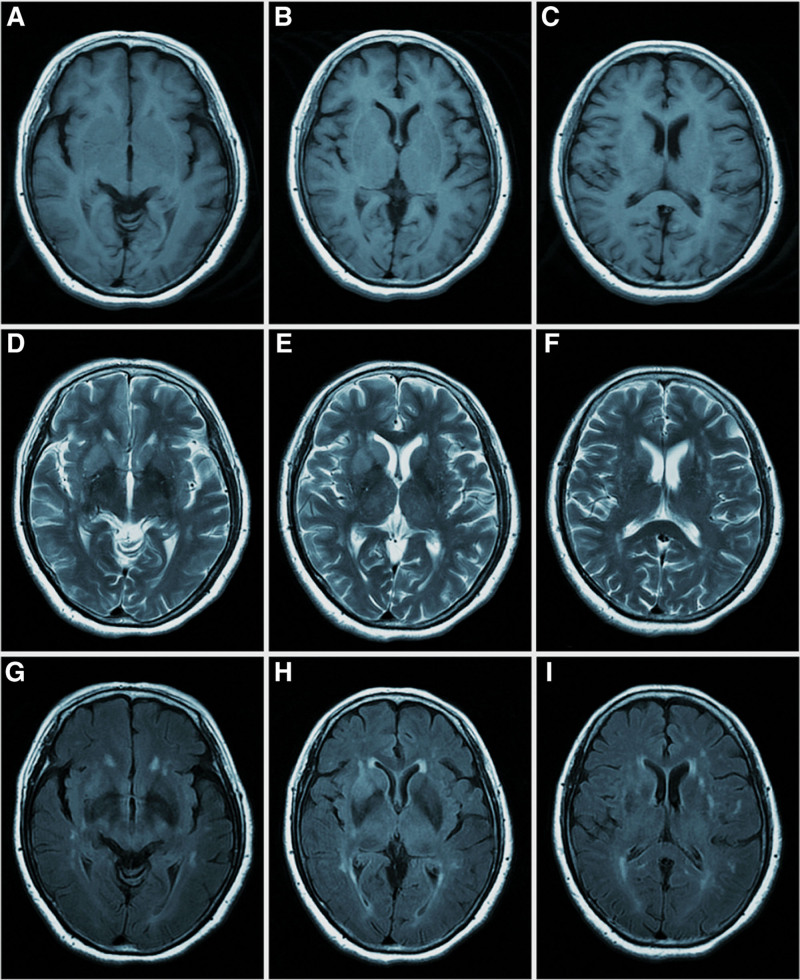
MR scan of the brain revealed abnormal signals in the right side of the caudate nucleus and putamen, with T1-weighted sequence of hypointensity (A–C), T2-weighted sequence of hyperintensity (E and F), FLAIR sequence of hyperintensity (G–I).
